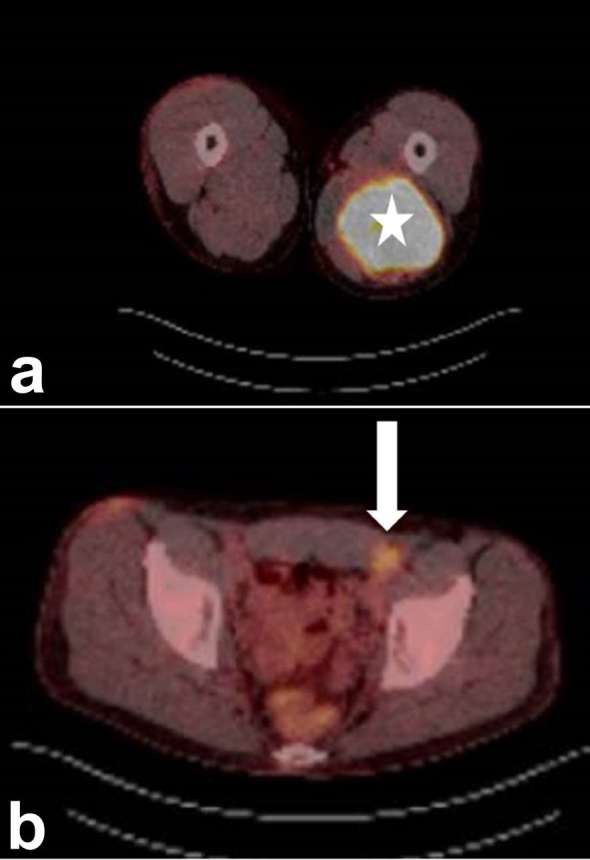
FDG PET/CT demonstrating intense radiotracer uptake (a) in the left thigh
